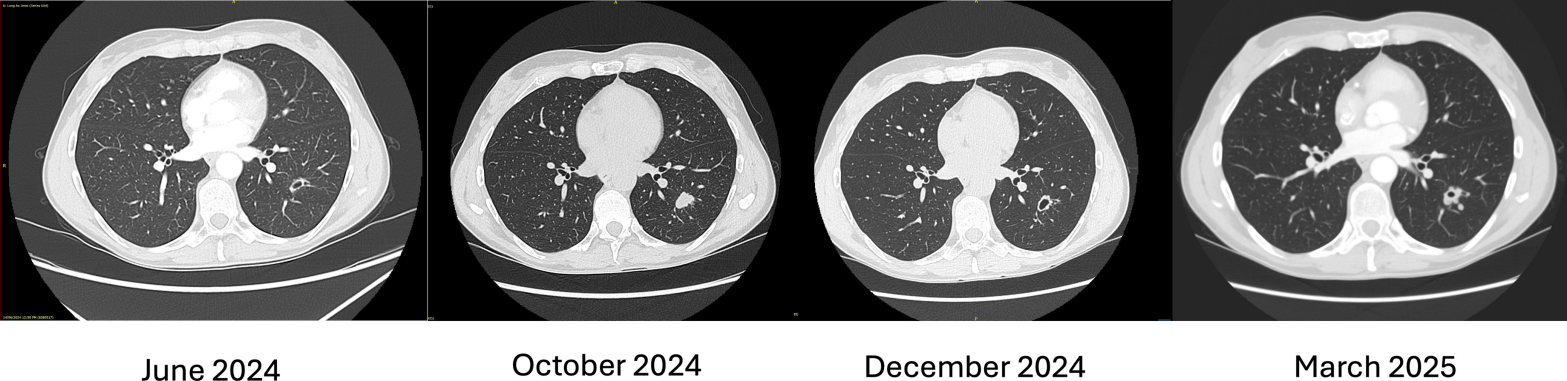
Serial CT Chest imaging taken June 2024, October 2024, December 2024 and March 2025.
